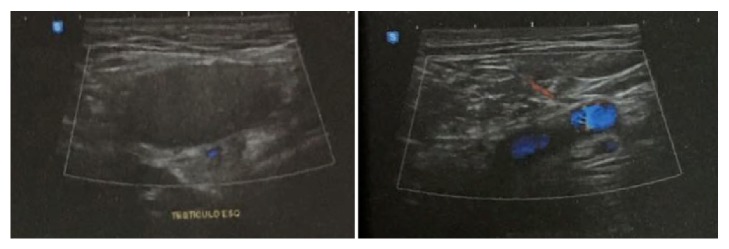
Doppler ultrasound of the left testicle.
